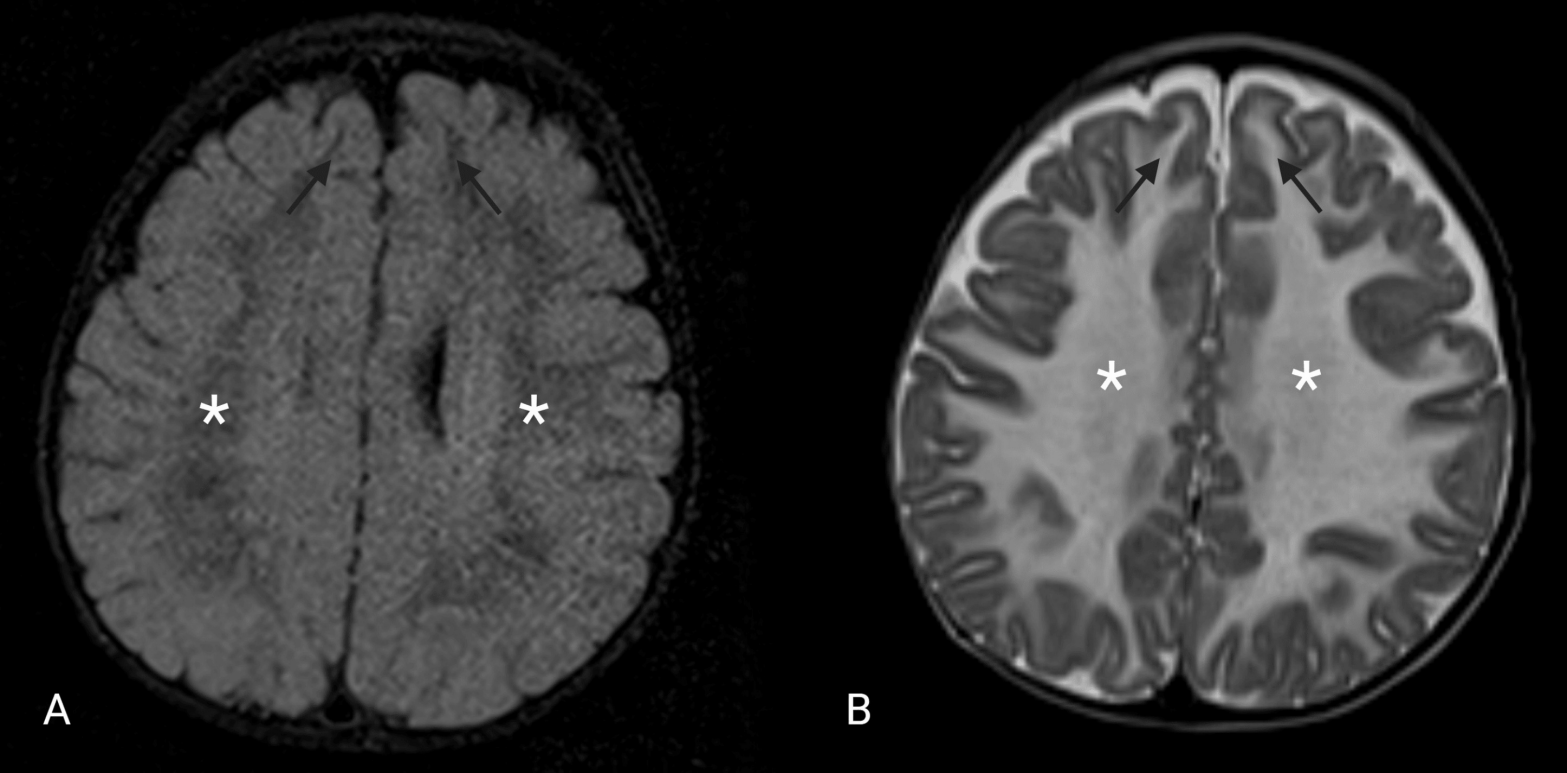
Supratentorial white matter rarefaction on T2 and FLAIR. (A) Axial fluid-attenuated inversion recovery (FLAIR) and (B) T2-weighted images at the frontoparietal level demonstrate diffuse, symmetric T2 hyperintensity involving the cerebral hemispheric white matter (white asterisks), including the subcortical white matter, without relative sparing of the subcortical U-fibers (black arrows). The deep white matter (white asterisks) shows corresponding marked FLAIR hypointensity. No definite signal abnormality is identified in the cerebral cortex.
